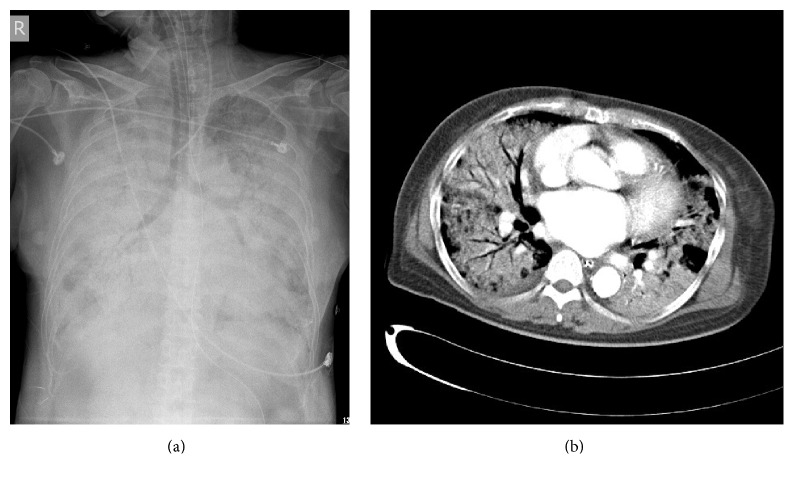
(a) A second chest X-ray showed bilateral consolidation with a snowflake pattern (8 h upon admission). (b) Chest computed tomography of the lungs demonstrated diffuse bilateral alveolar and interstitial infiltrations with a presentation compatible with acute respiratory distress syndrome (ARDS).
